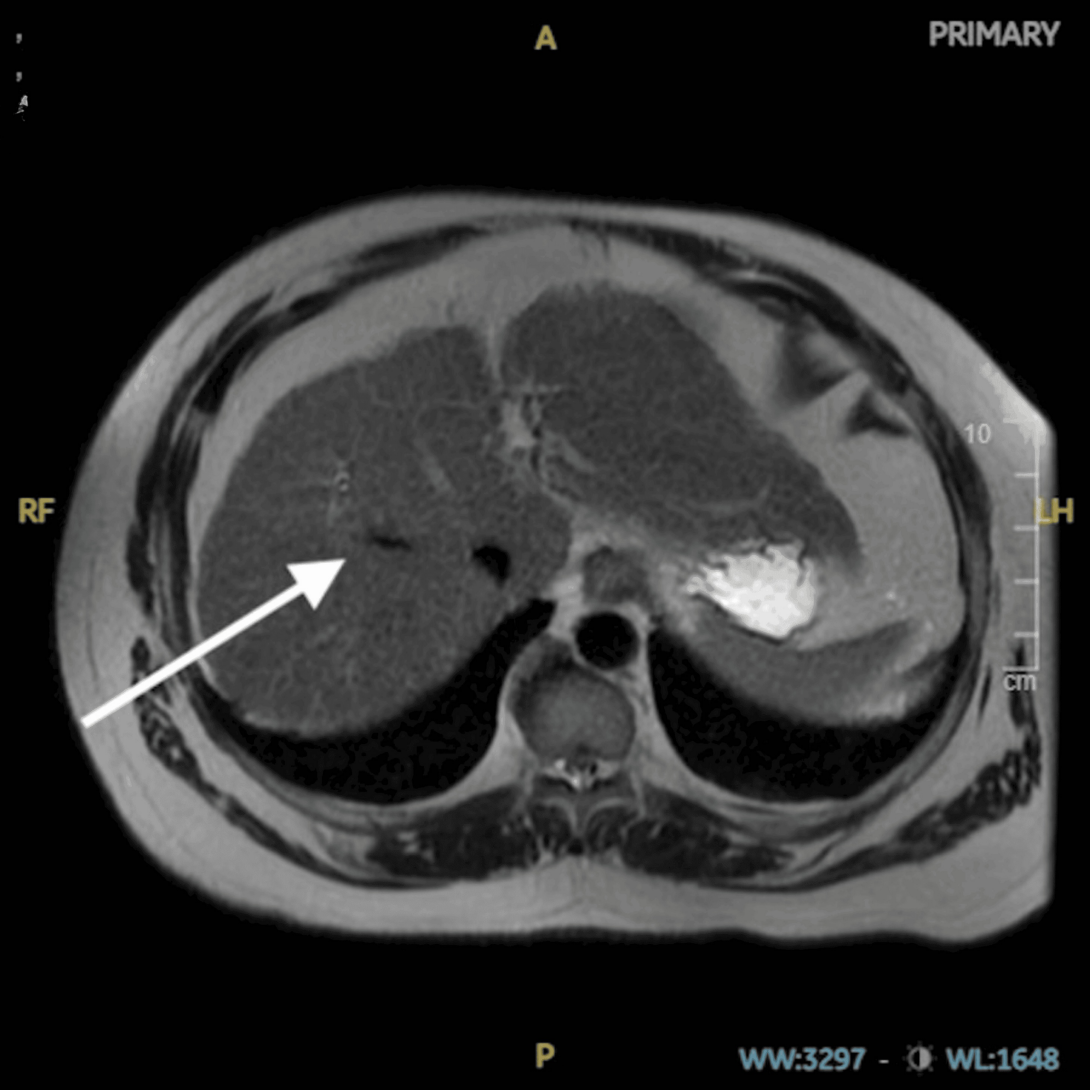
MRI showing liver echogenicity MRI: magnetic resonance imaging
